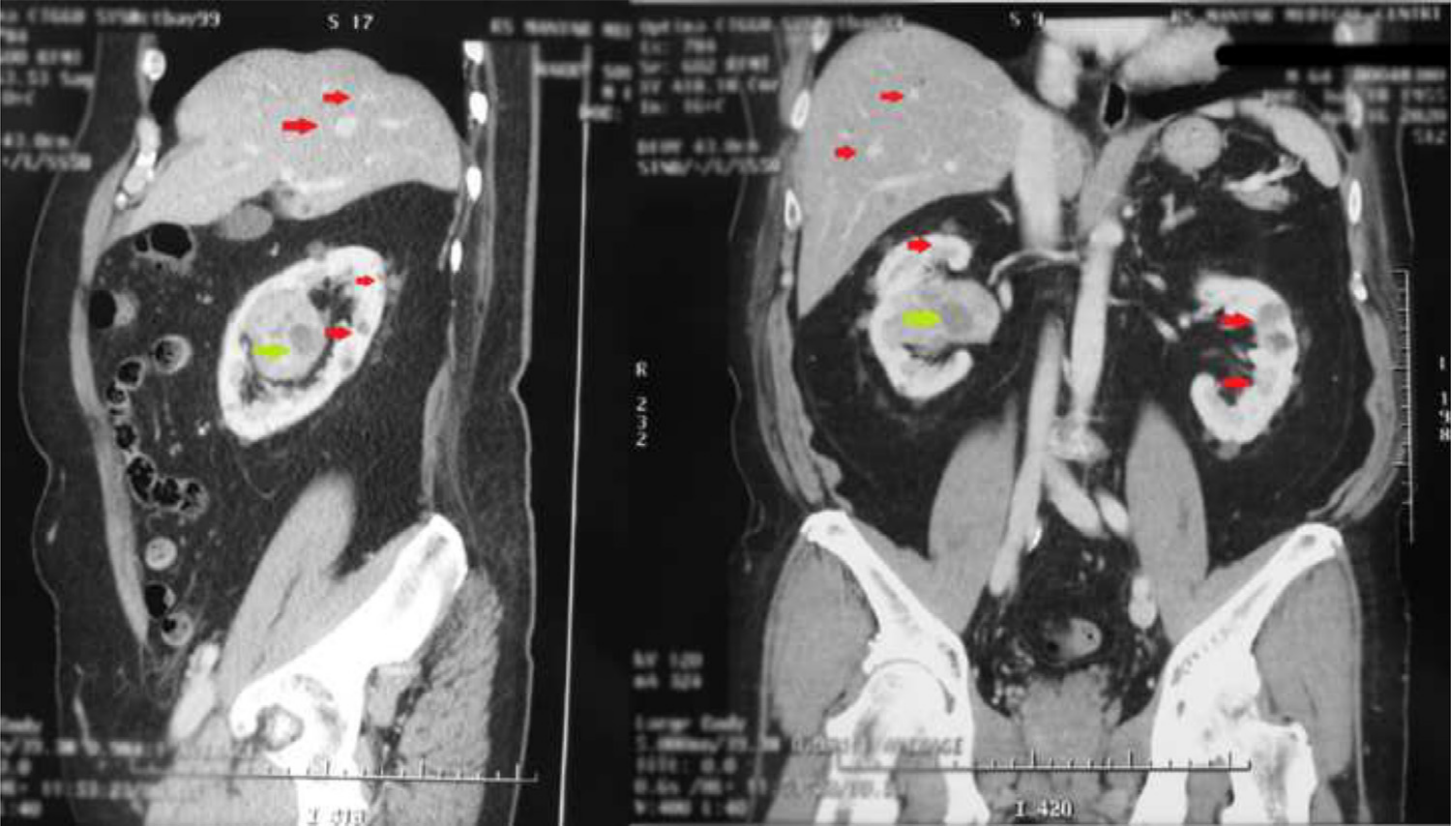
The results of the CT-IVP examination revealed a lobulated heterogeneous mass in the upper to middle pole of the right kidney, accompanied by multiple cysts on the right and left kidneys (green arrow). Multiple cysts were found in the right and left lobes of the liver (red arrow).
